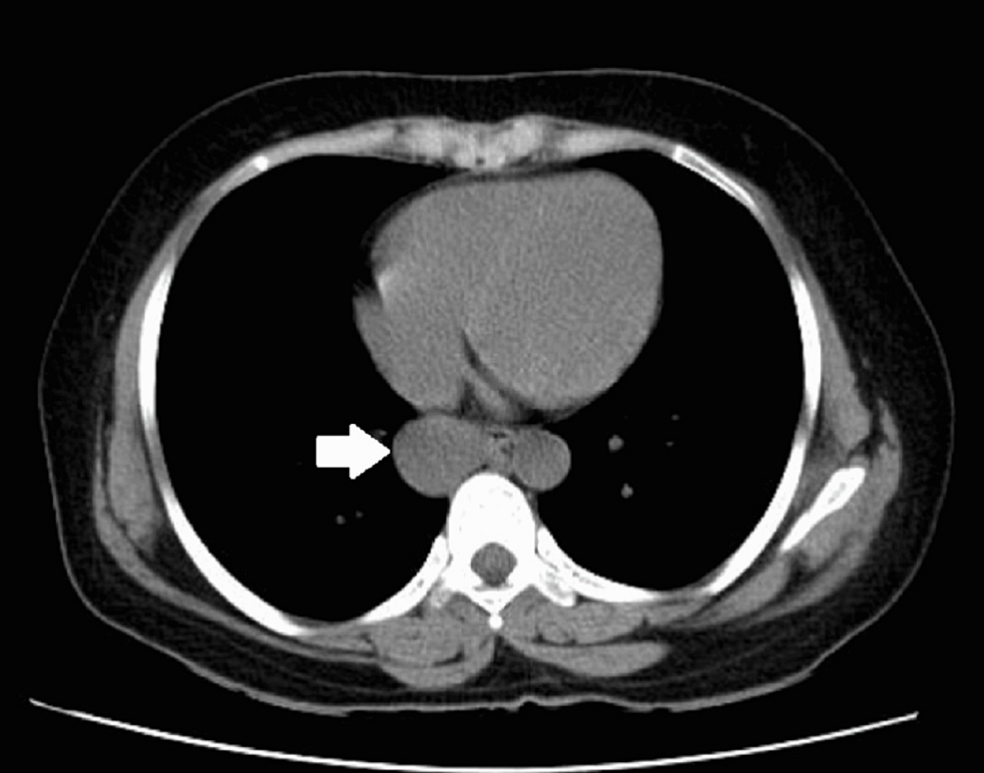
Axial CT of the chest showing a well-defined hypoattenuating lesion in the posterior mediastinum (arrow)
